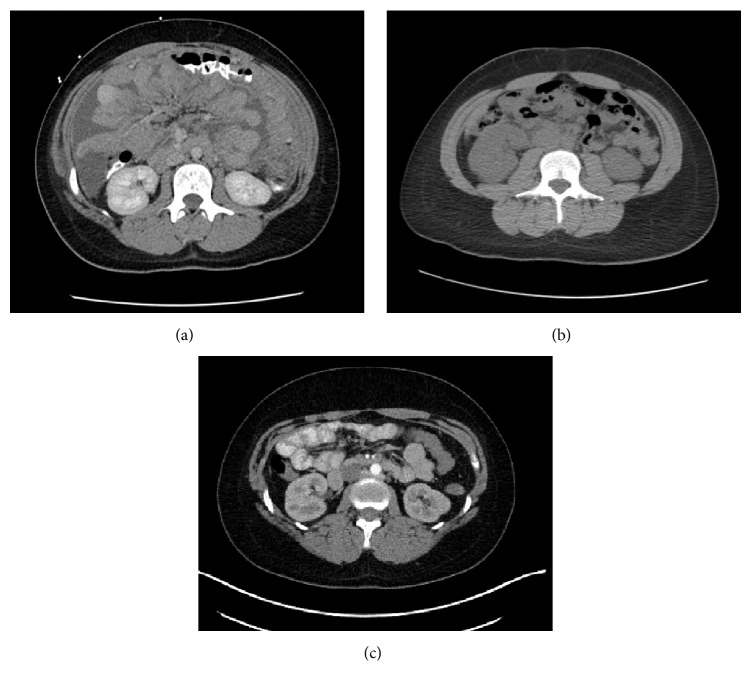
Contrast enhanced CT, axial view, mid abdomen. (a) Completed October 2016 with benign findings. (b) Completed November 2016 with extensive thickening, nodularity, and enhancement of the peritoneum and omentum. (c) Completed June 2017 with benign findings.
